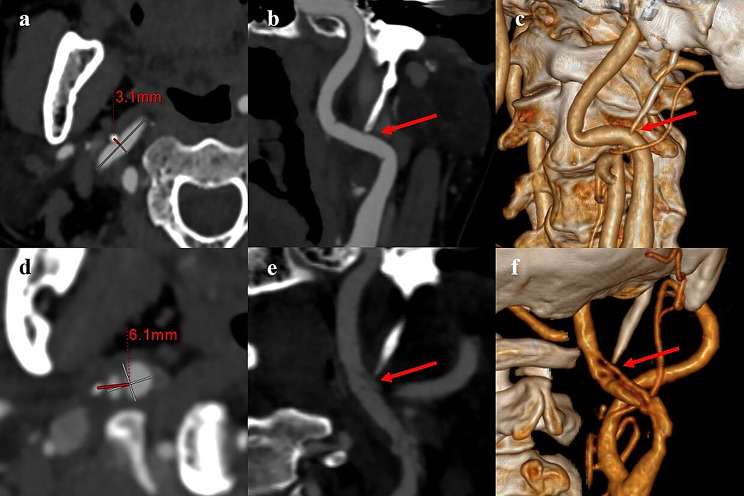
Measurement of the SPT–ICA distance and spatial relationship between the SP tip and the ICA on CTA. a The distance from the SP tip to the geometric center of the ICA lumen (red line). b-c The spatial relationship between the SP tip and the ICA in the non-dissection case (red arrow). d At the SP tip level, the distance is taken from the SP tip to the geometric center of the dissected-artery complex (red line). e-f The spatial relationship between the SP tip and the ICA in the dissection case (red arrow)
